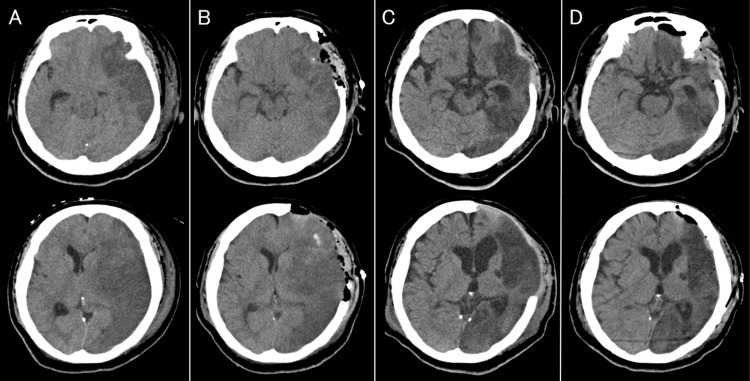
Serial changes on head CT. Day 1 CT demonstrating extensive infarction in the left middle cerebral artery territory with midline shift and uncal herniation (A). CT after decompressive craniectomy on Day 2 showing improvement of midline shift and resolution of uncal herniation (B). CT on Day 40 demonstrating resolution of cerebral edema (C). CT after cranioplasty on Day 44 (D).
